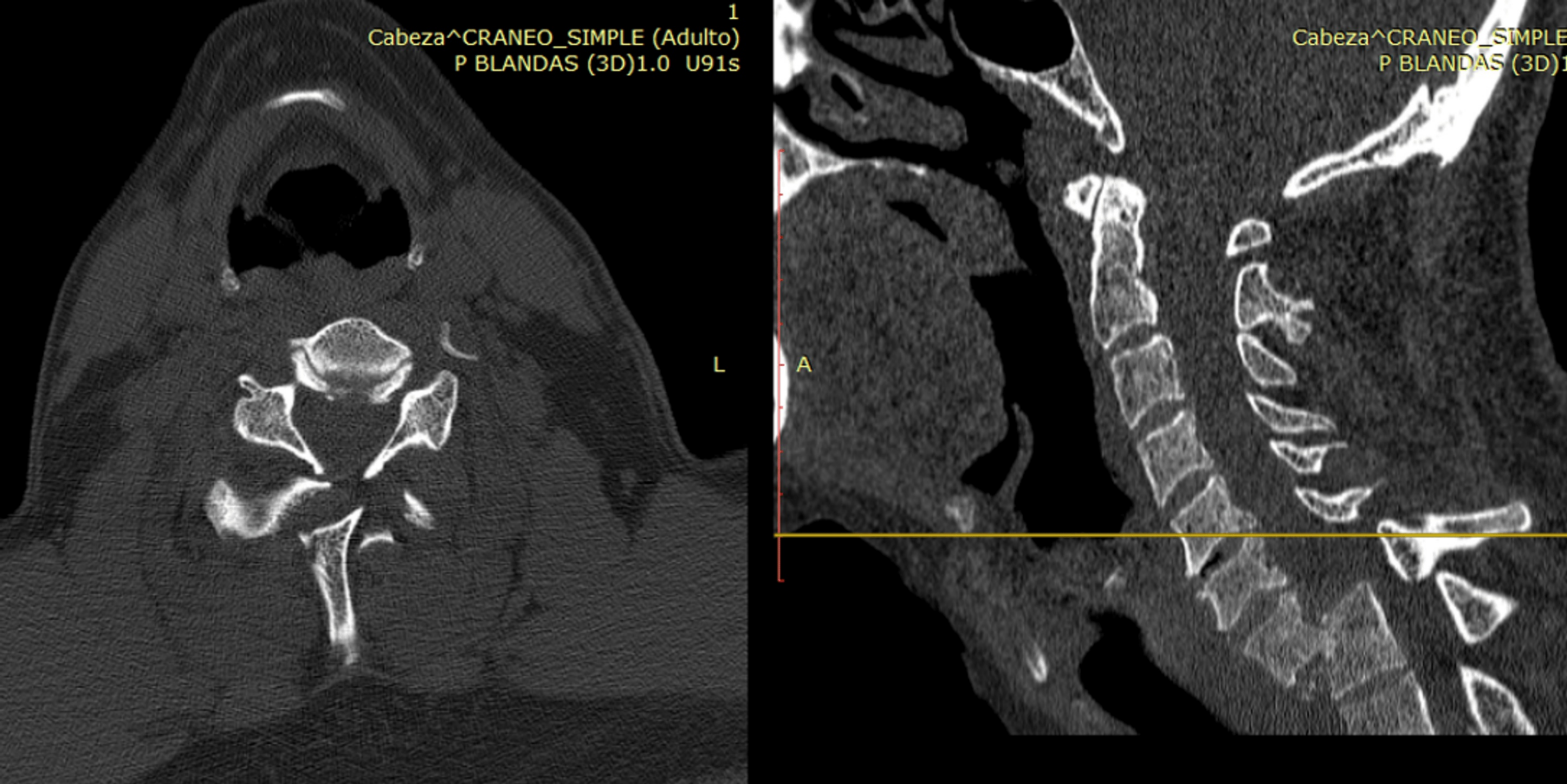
Case 4 CT scan axial and sagittal slices showing the injury of the C7 vertebral body over T1.
